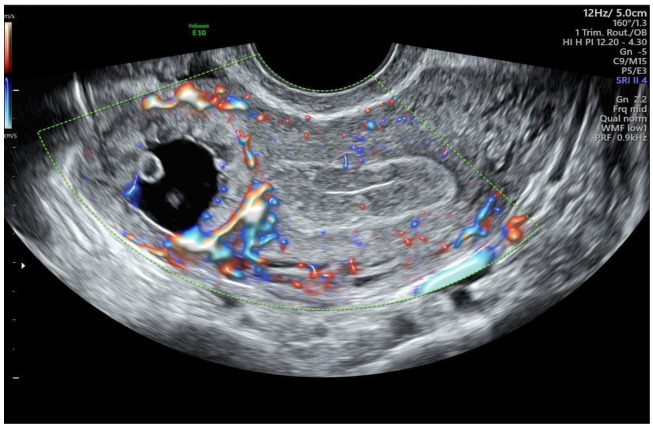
Transvaginal ultrasound finding of interstitial pregnancy.
